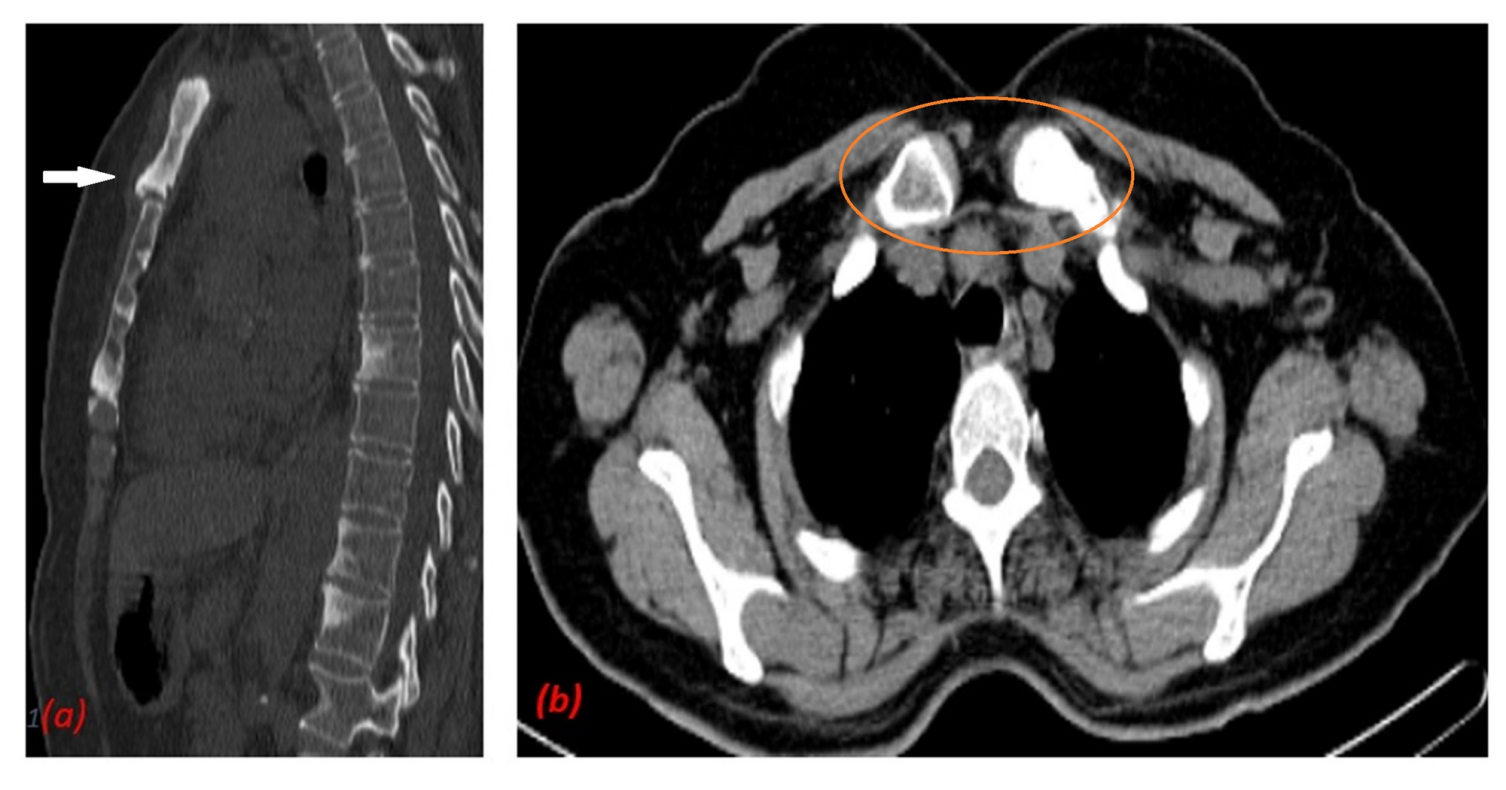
(a) CT scan of the chest shows sclerosis of manubrium (white arrow) and (b) sclerosis of medial part of first ribs (orange circle).
